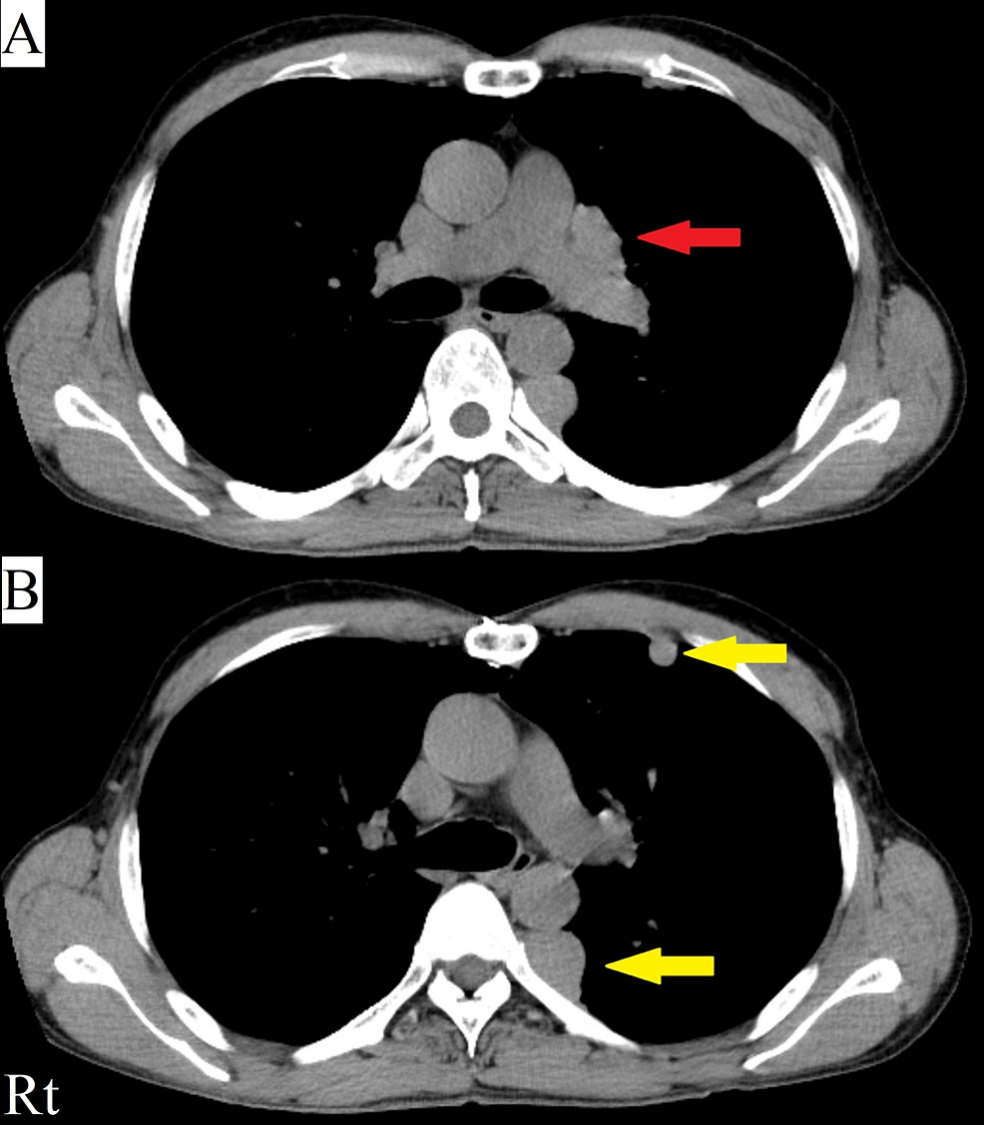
Transverse sections on CT (soft tissue window setting) demonstrating metastatic thymoma in the patient in Case 1. (A) Invasive thymoma metastasis to the mediastinal region (red arrow). (B) Invasive thymoma metastasis to the lateral pleura (yellow arrows). CT, computed tomography
